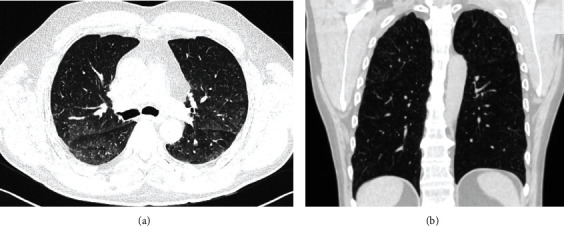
High-resolution computed tomography (HRCT) images (a, b) demonstrate diffuse, multiple nodular lesions in the parenchyma of both lungs.
